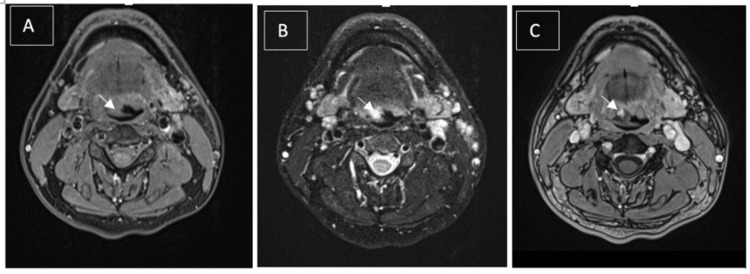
(A) T1-weighted axial MRI neck, (B) T2-weighted axial MRI neck, and (C) T1-weighted axial MRI neck (post-contrast) (A, B, C): A well-defined lesion is observed at the right base of the tongue, appearing homogeneously isointense on T1 and hyperintense on T2. It does not involve the extrinsic muscles of the tongue but abuts the vallecula. Post-contrast images show incomplete enhancement of the lesion at the right tongue base, with no arterial supply to the lesion. MRI: magnetic resonance imaging
